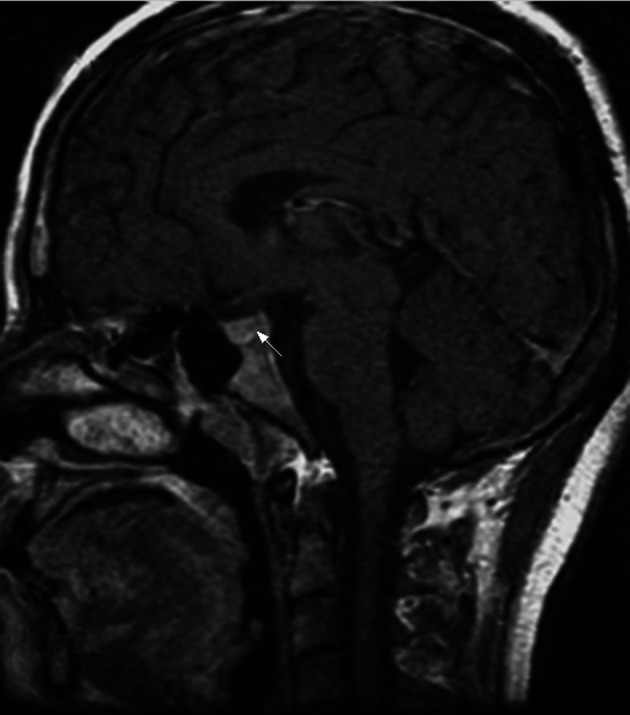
Non-enhanced magnetic resonance image (MRI) of the sagittal section of patient 2 showing the disappearance of the characteristic high signals of the posterior pituitary and increased size of the pituitary stalk.
